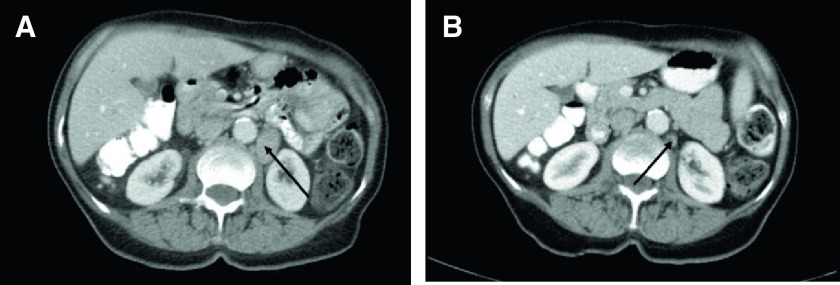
Computed tomography scans for case 1 (A) before (June 2016) and (B) after (May 2017) starting vemurafenib. Arrows indicate decrease in size of lymph node.
